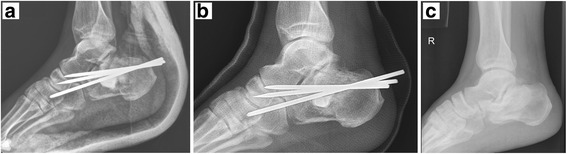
Calcaneal fracture in a 61-year-old man (continued). a A postoperative plain film demonstrating restoration of Böhler’s angle. b A postoperative plain film two months postoperatively. c A reduction of Böhler’s angle was noted 20 months later
